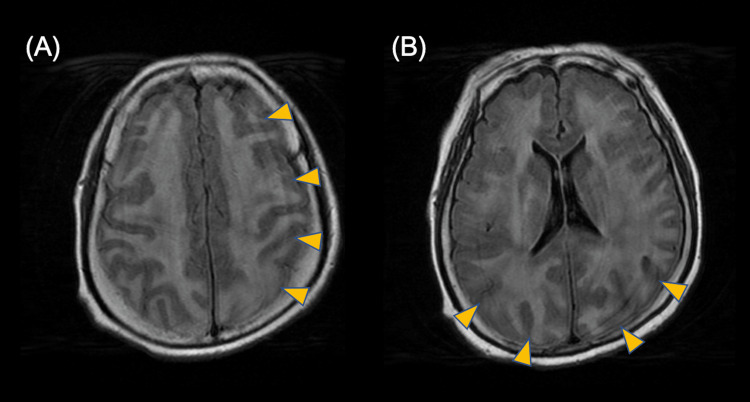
Head magnetic resonance imaging shows symmetrical and bright appearance lesions predominantly in the (A) frontal, parietal, and (B) occipital lobes (fluid-attenuated inversion recovery image sequence).
